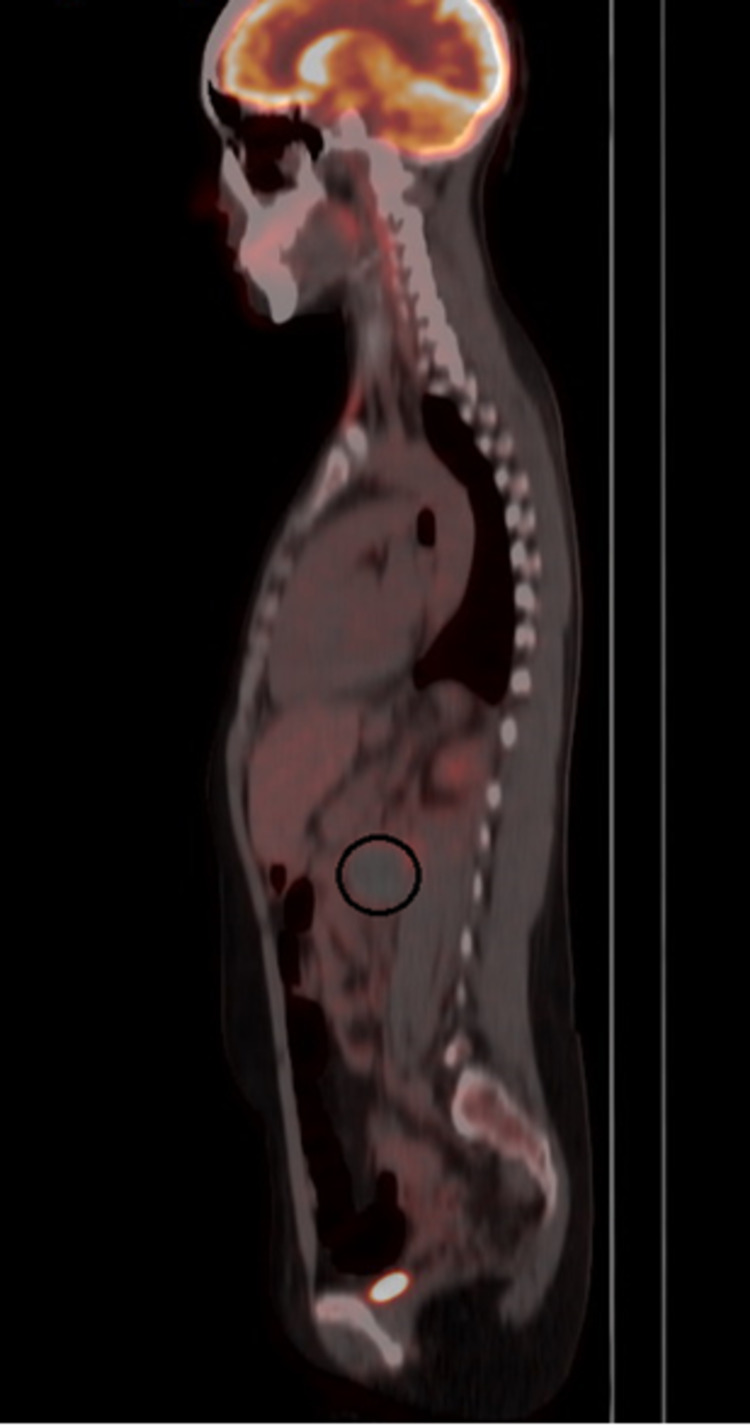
PET/CT with 18F-fluorodeoxyglucose (sagittal section) The left para-aortic oval image described in Figure 1 is described in a sagittal section highlighted with a black circle. PET: positron emission tomography
